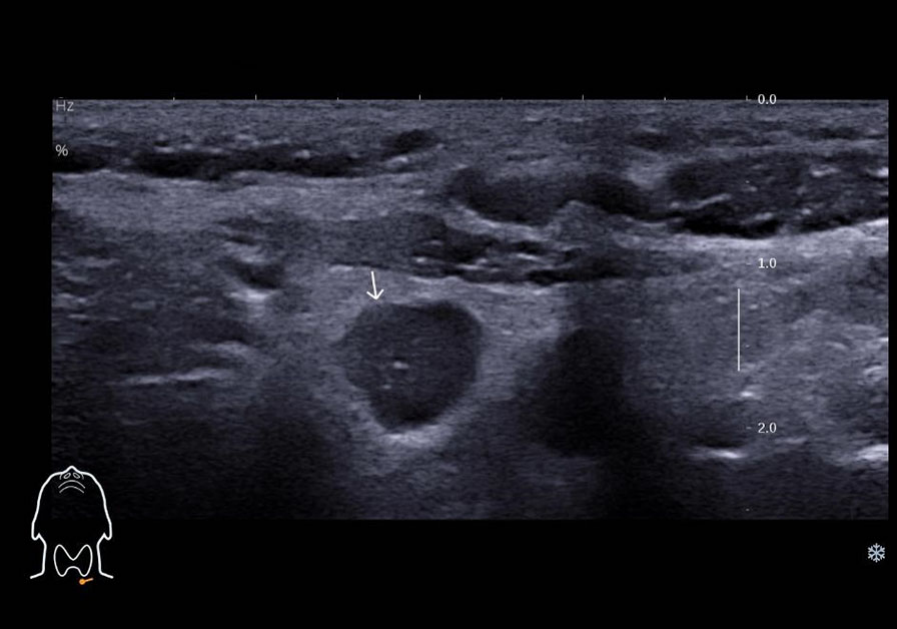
Ultrasound image depicting lymph node metastasis of medullary thyroid carcinoma in cervical region VI, demonstrating internal fine calcifications.
